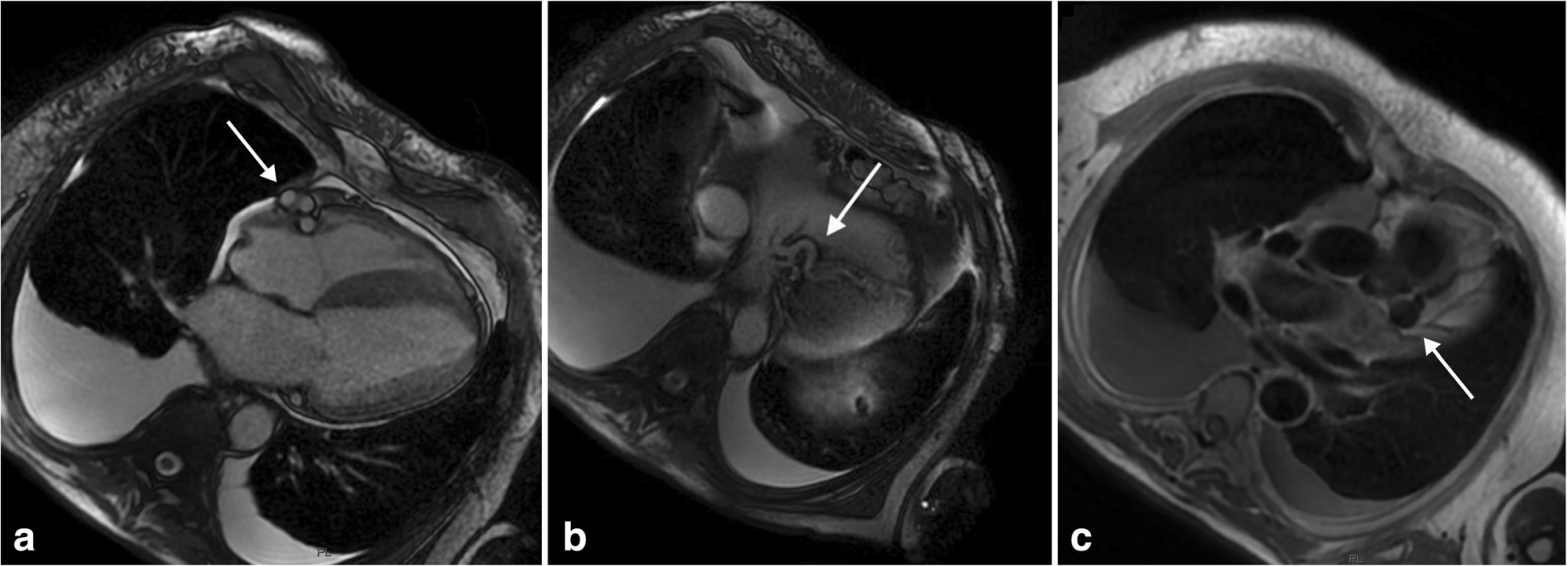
a and b Cardiac magnetic resonance imaging steady-state free precession cine sequence, four-chamber plane, showing a plausible coronary artery anomaly: dilated and tortuous right coronary artery (*arrow* in a), probably anastomosed with the distal portion of the left anterior descending artery (*arrow* in b). c T1-weighted black-blood sequence demonstrating a left coronary aneurysm (*arrow* in c), a feature suggestive of a coronary anomaly
